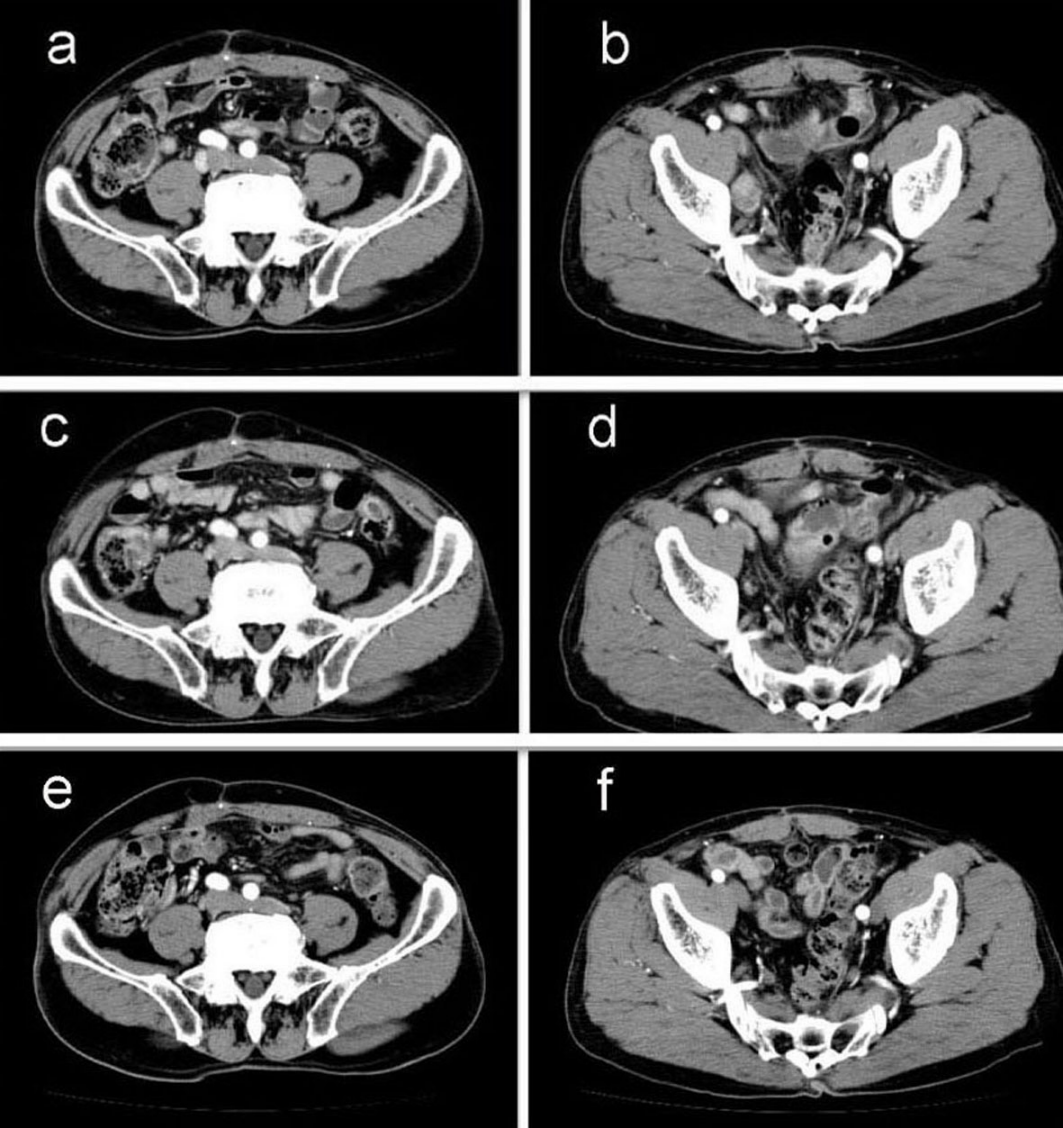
Abdominal computed tomography (CT) findings. a-b): CT scan before chemotherapy showing swollen para-aortic and right lateral pelvic lymph nodes; c-d): CT scan after 5 cycles of S-1 administration showing reduction in the swollen para-aortic lymph nodes; e-f): CT scan after radiation and 10 cycles of S-1 administration showing complete disappearance of swollen para-aortic and lateral pelvic lymph nodes. Hence, response in this case was classified as a complete response.
